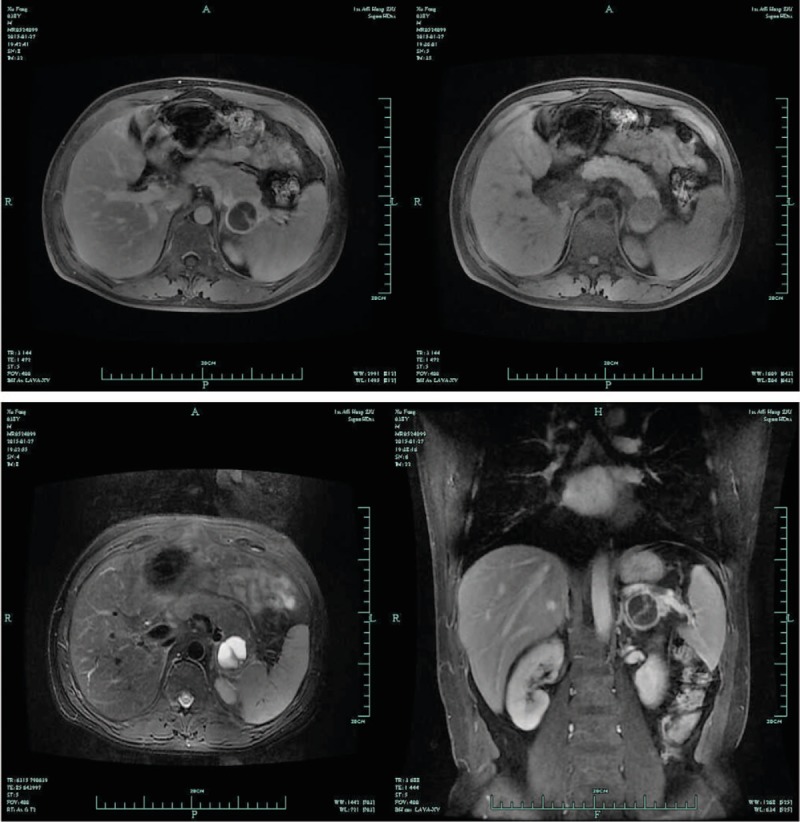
A cystic lesion in tail of pancreas presented lower intensity on T1-weighed MRI and higher intensity on T2 MRI study.
